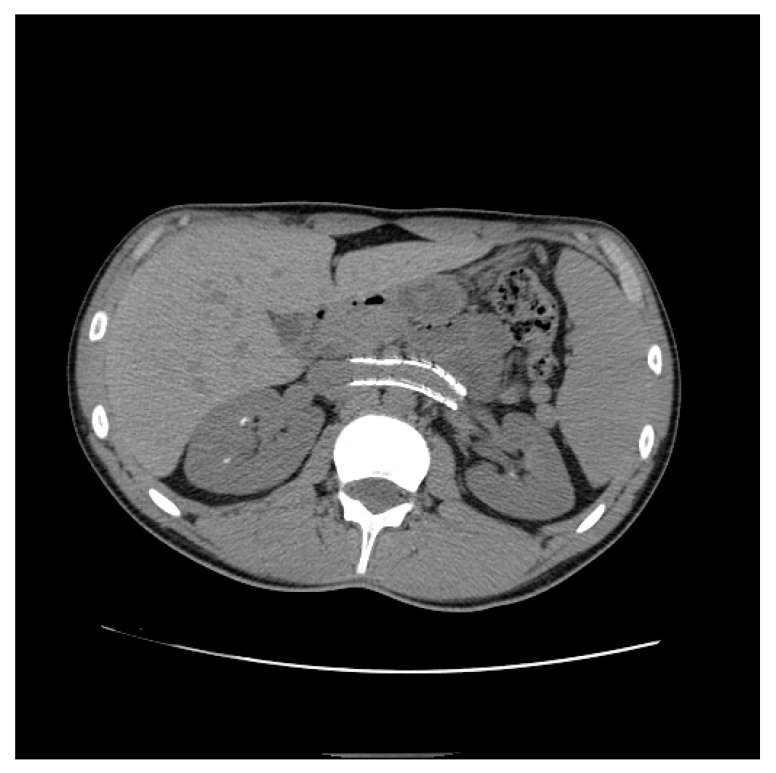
Patient who previously underwent endovascular treatment with symptom resolution, subjected to imaging investigations for other reasons. Non-contrast axial CT passing through aorto-mesenteric clamp demonstrated the correct positioning of a stent in the left renal vein with resolution of the stenosis.
